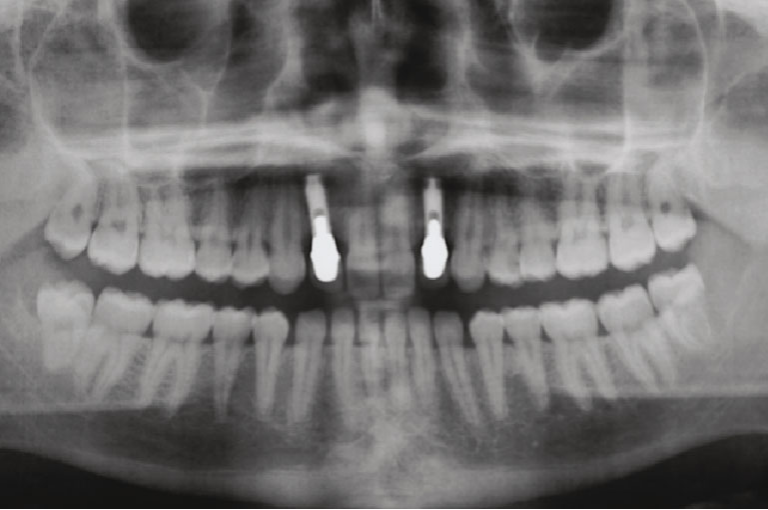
Orthopantomography at 8 years.
